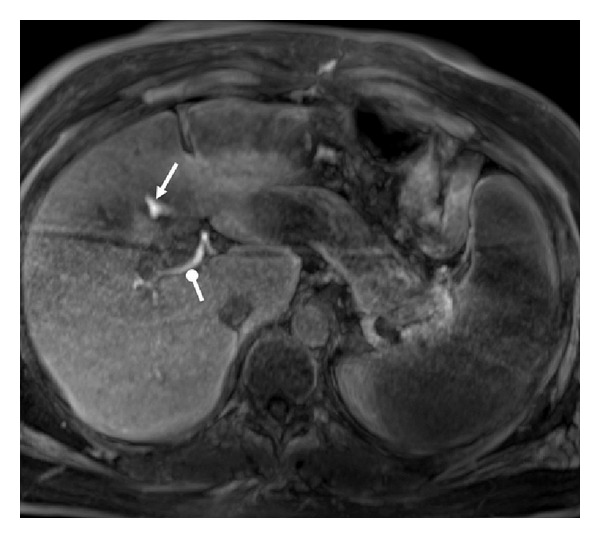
Magnetic resonance imaging showing bile leakage.* Subvolume rendering of T1-weighted fat suppressed axial gradient echo acquisition approximately 45 minutes after intravenous administration of Gd-EOB-DTPA (Primovist; Bayer-Schering, Berlin, Germany) showing contrast-enhanced bile in central intrahepatic bile ducts (dot-head) and verifying bile leakage in gallbladder fossa (arrow).
